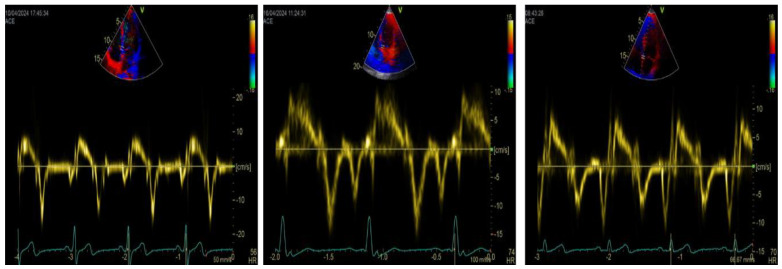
Age and diastolic velocities by tissue Doppler imaging (TDI). Septal TDI traces measured in three healthy male individuals (aged 17, 33, and 62 years) are reported to show the inverse behaviors of early (e’) and late (a’) diastolic TDI velocities across different ages. At 17 years, TDI-e’ and a’ were16 and 6 cm/s, respectively (left panel); at 33 years (mid panel), TDI-e’ and a’ were 11 and 9 cm/s, respectively; at 62 years (right panel), TDI-e’ and a’ were 8 and 11 cm/s, respectively.
